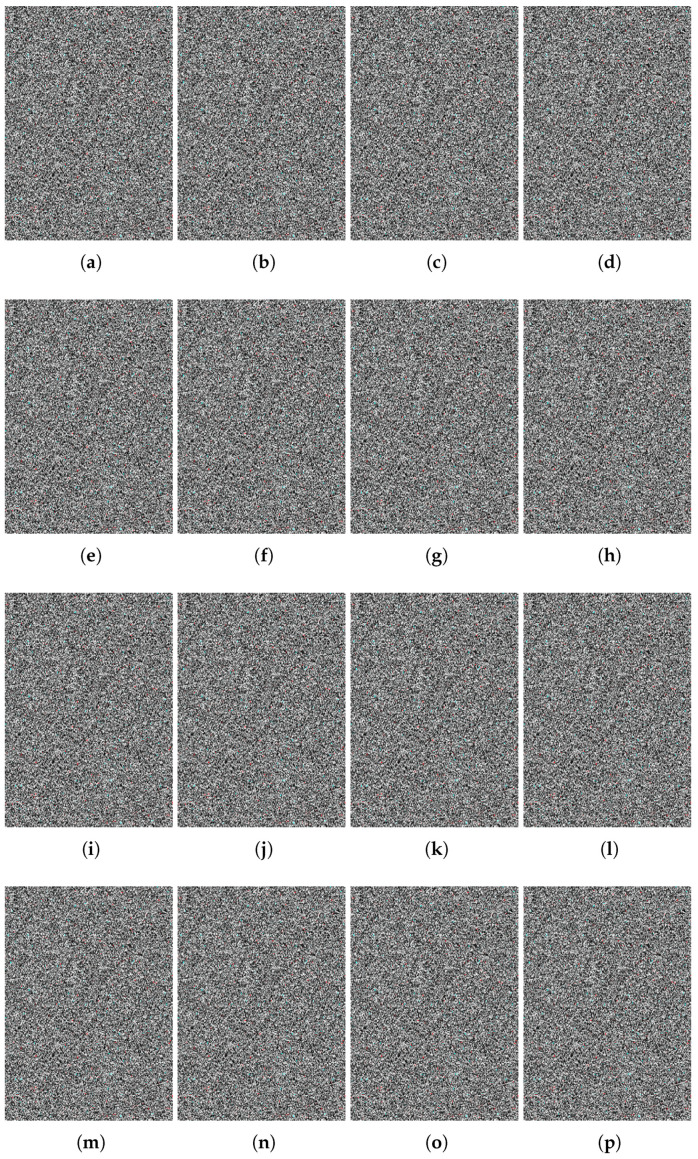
(a–p) Encrypted images corresponding to normal and pneumonia patients’ X-ray images.
